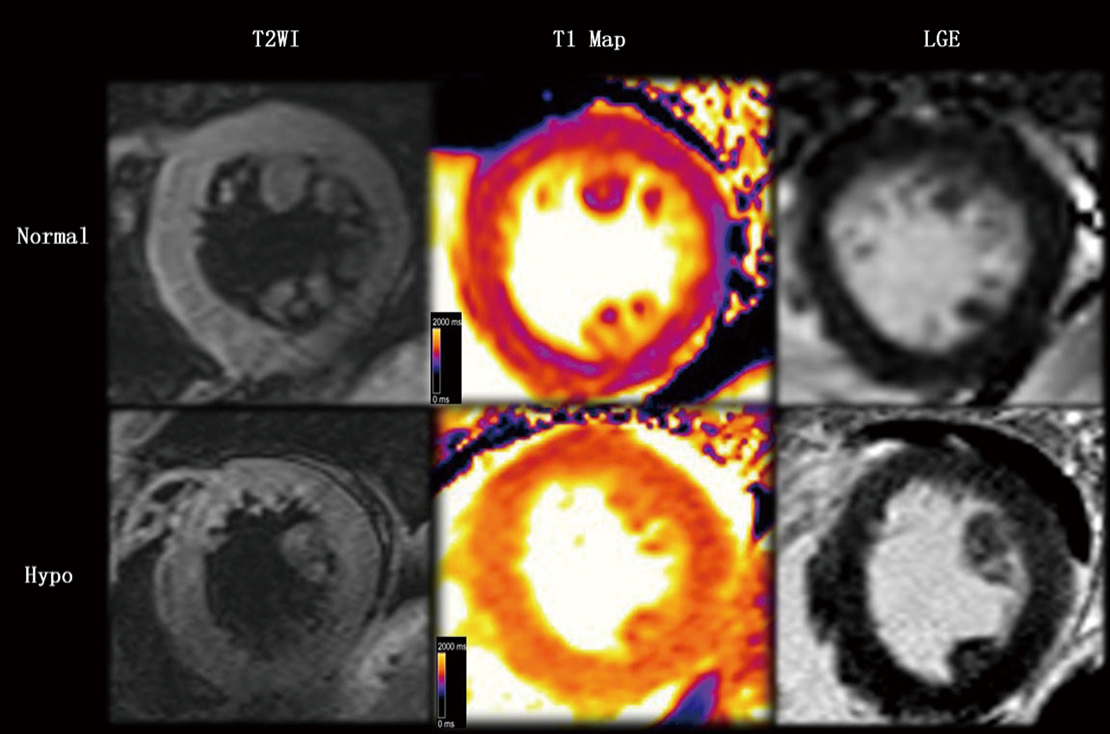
Representative cardiac magnetic resonance images from a 42-year-old patient with overt hypothyroidism (Hypo) and normal control (Normal). (Left) T2WI showed a homogenous intensity within the left ventricular and overt pericardial effusion in Hypo. (Middle) color T1 maps based on a native modified Look-Locker inversion. Note the markedly elevated myocardial T1 time in the Hypo (T1 = 1301 ms, orange range of the color scale) compared with the Normal control (T1 = 1040 ms, purple range of the scale). (Right) on LGE, myocardial had a homogenous intensity in Hypo which was similar to intensity in Normal control.
